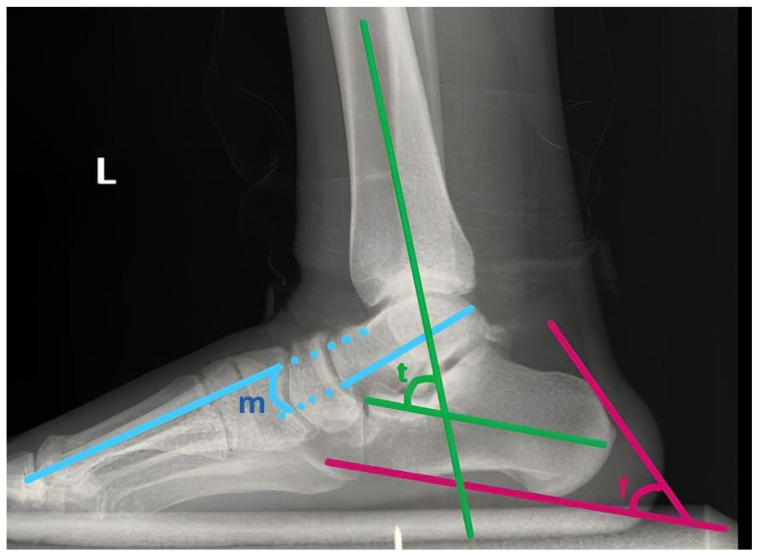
Radiographic measurement of foot. (f: Fowler and Flippe angle, m: Meary angle, t: Tibiocalcaneal angle).
